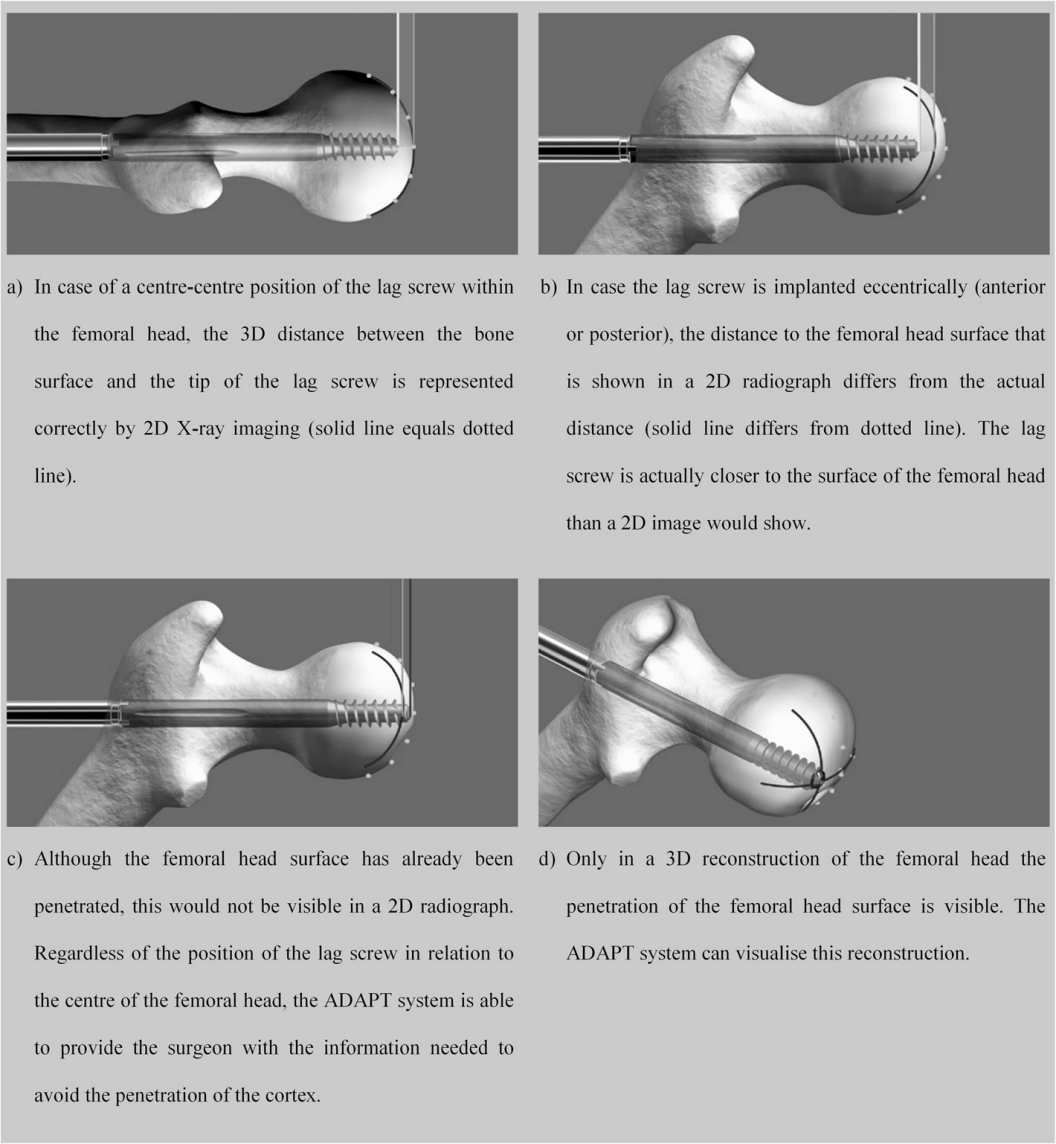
Difference between 2D and 3D image information; screenshots taken from FluoroMap software (solid lines represent the real surface of the femoral head based on 3D information, dotted lines represent the outline of the femoral head as shown in 2D radiographs). (a) Congruence of the visible and actual 3D distance to the bone surface in case of a centric lag screw placement, (b) lack of congruence of the visible and actual 3D distance to the bone surface in case of an eccentric lag screw placement, (c) penetration of the femoral head surface despite inconspicuous 2D imaging, (d) 3D reconstruction of the femoral head revealing the penetration of the femoral head surface.
